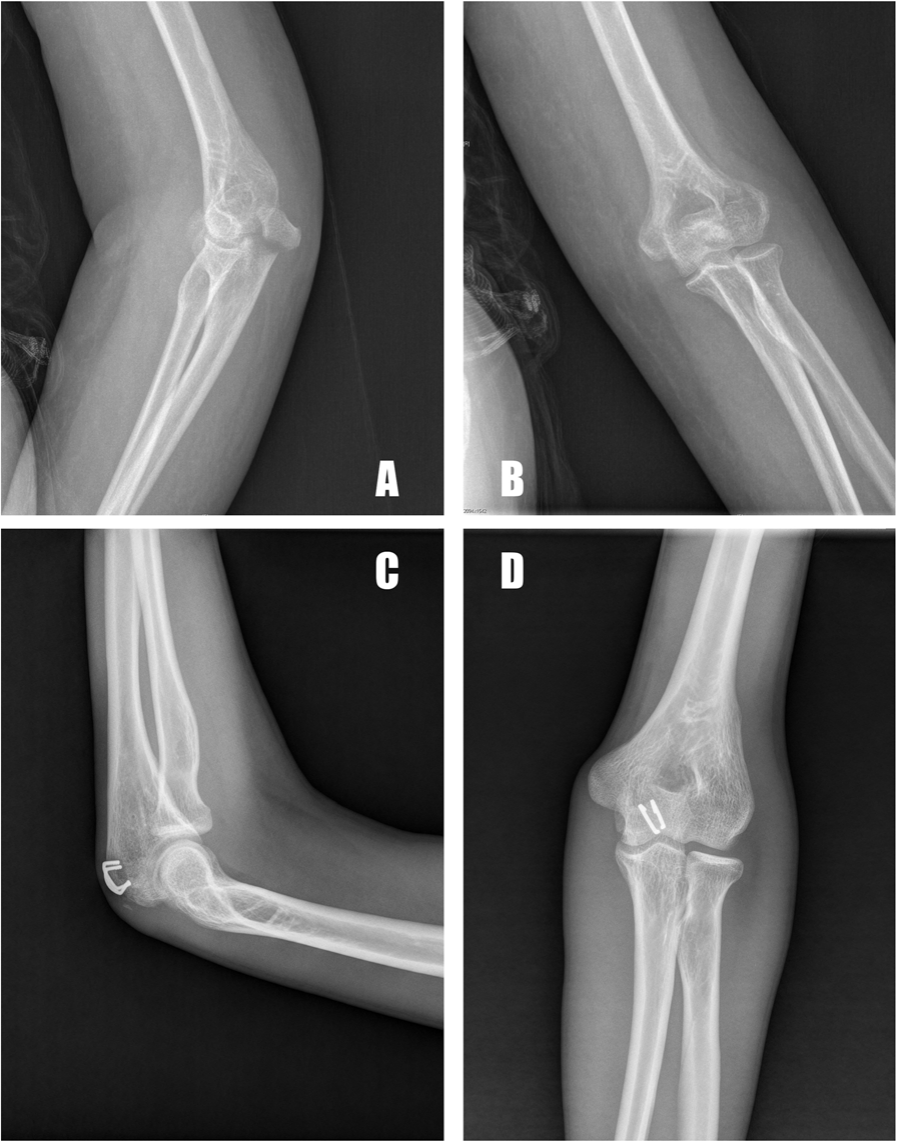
A 38-year-old male patient with olecranon fracture caused by a fall onto his ankle (a, b). Fragments were reduced and fixed with the ASC. Ten months after the surgery, the radiographs showed a healed fracture and the ankle joint surface was anatomically reduced without any gap or step-off (c, d)
